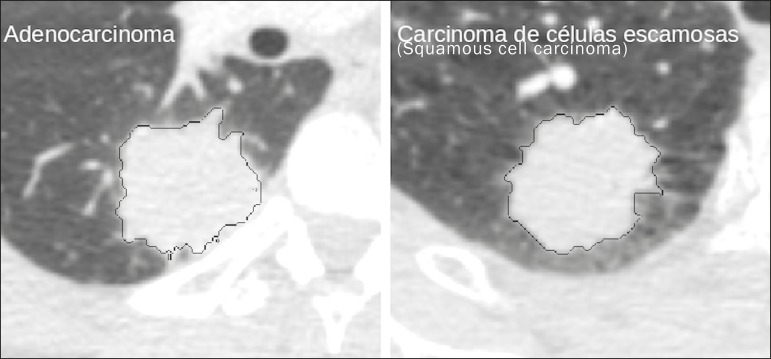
Segmentation of pulmonary nodules on chest CT in two different patients, both nodules having irregular contours and being in proximity with adjacent vessels or chest wall, factors that make it difficult to segment the edges of lesions properly. In such cases, texture analysis can facilitate proper segmentation.
